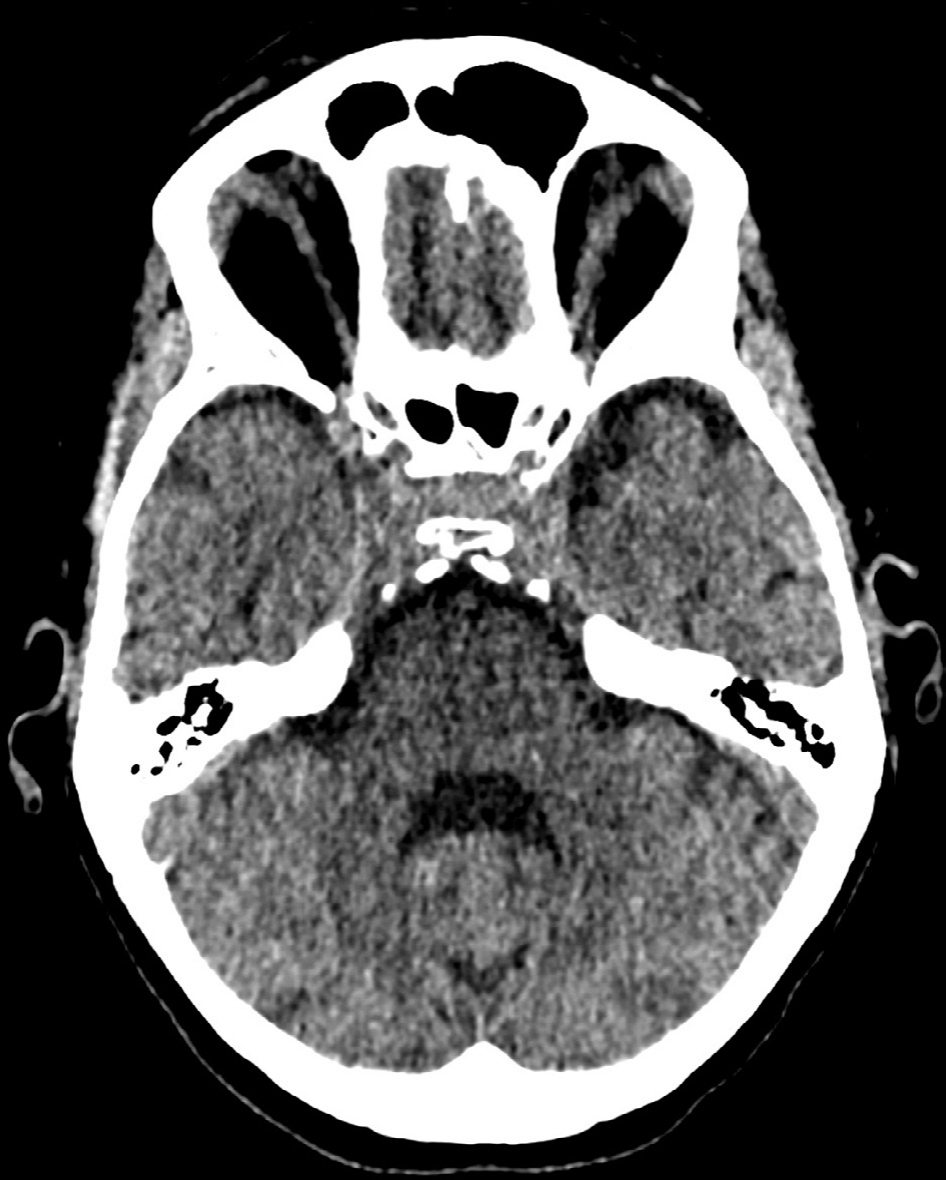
Axial, non-contrast CT image at the same level, at the level of the pons.
